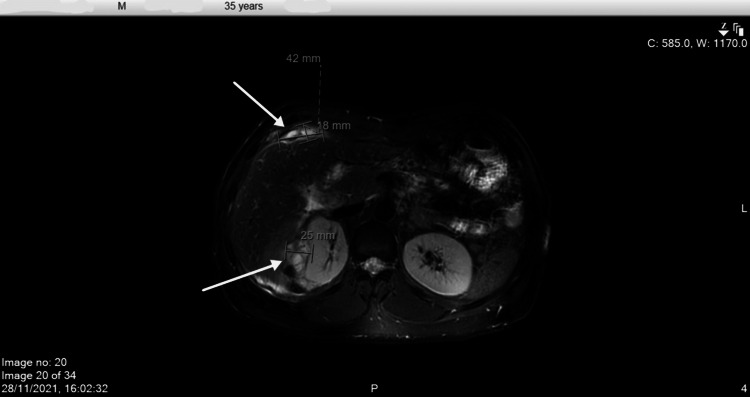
MRI abdomen/pelvic (axial plane) The image shows additional similar perihepatic nodules overlying the left hepatic lobe anteriorly and posteriorly. MRI, magnetic resonance imaging.
